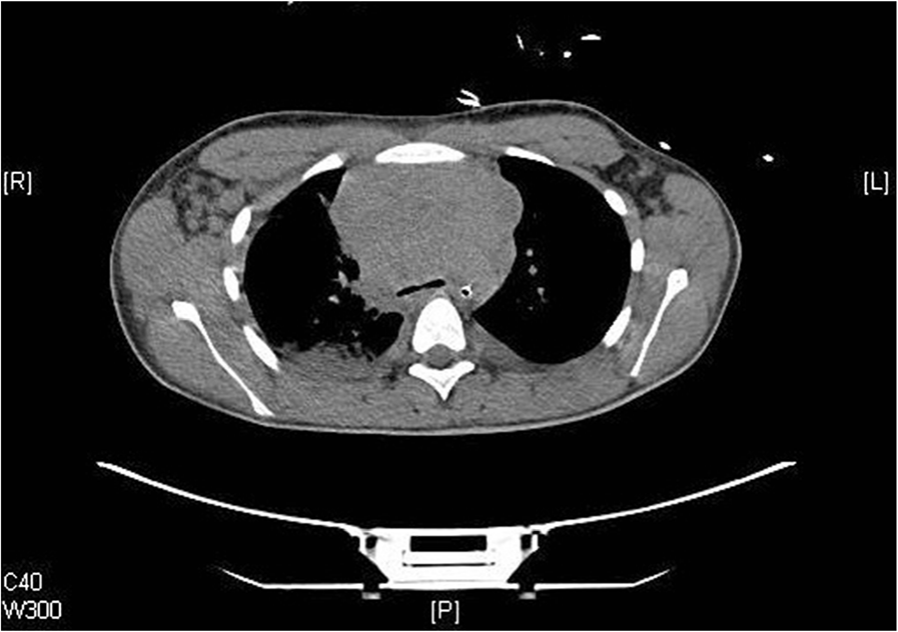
Computed tomography scan of mediastinum. Massive mediastinal tumor mass.
